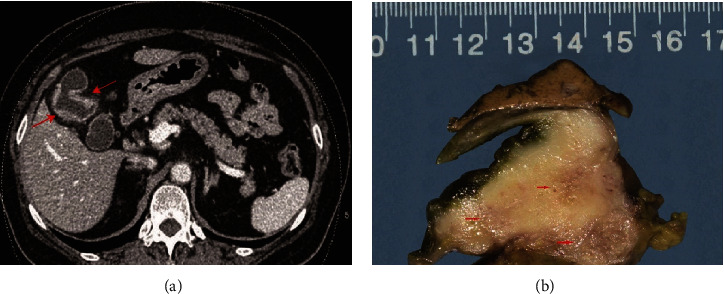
(a) An abdominal contrast-enhanced CT scan showing diffuse thickening of the gallbladder wall, with significant enhancement (arrows) in the portal venous phase. (b) Gross image of the gallbladder showing a thickened wall; the cut surface shows a tan-white thickened submucosal layer (arrows) invading through the wall into the surrounding adipose tissue, which did not invade the adjacent liver parenchyma.
